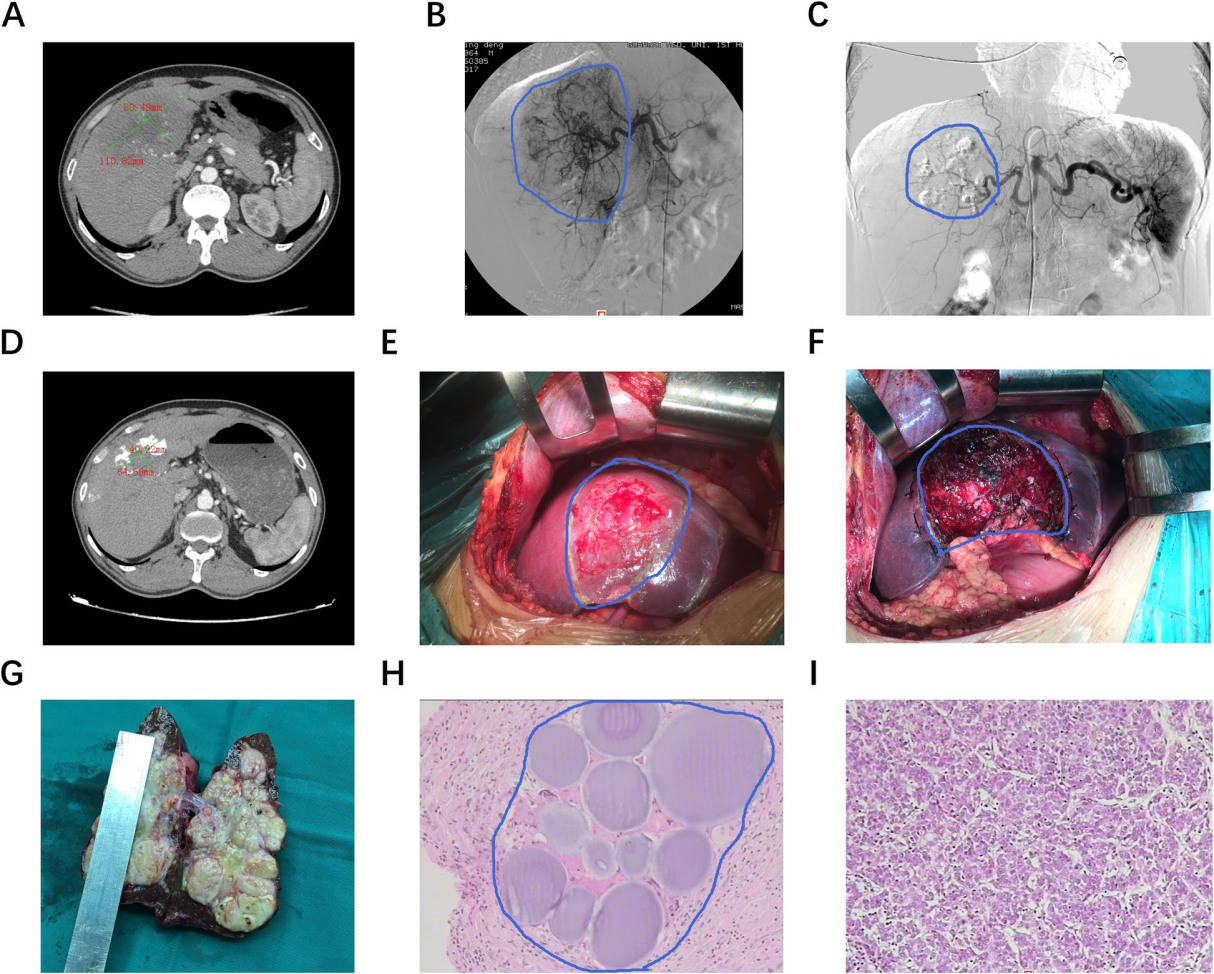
Typical case report. A Representative images of tumor by abdominal computed tomography scan before DEB-TACE treatment. B Representative images of DEB-TACE treatment. C Representative images of hepatic arteriography during DEB-TACE. D Representative images of tumor abdominal computed tomography scan after DEB-TACE treatment. E–F Representative images of tumor during resection. G Representative images of resected tumor. H–I Representative images of tumor pathological examination
